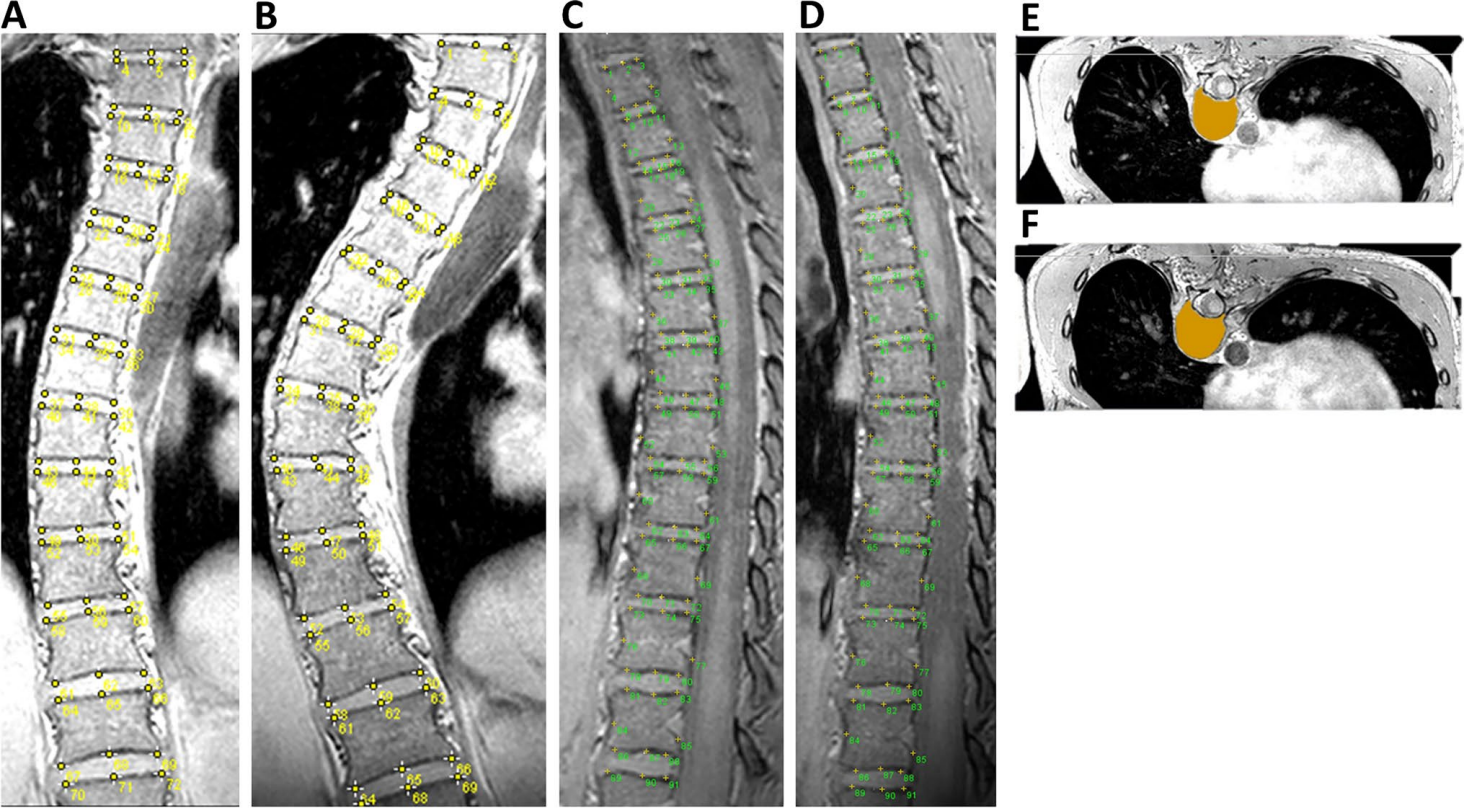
Orthogonal MRI reformatting images from final MRI scans of a non-progressive AIS curve (coronal Cobb angle = 23 deg; major thoracic kyphosis angle = 5 deg; rib hump = 13 deg) (Images A, C and E, respectively), and a progressive AIS curve (coronal Cobb angle = 64 deg; major thoracic kyphosis angle = 13 deg; rib hump = 18 deg) (Images B, D and F, respectively). Images A and B highlight coronal plane segmental anatomic markers. Images C and D highlight sagittal plane segmental anatomic markers. Images E and F highlight axial plane cuts at the curves’ apices, with an overlying annotation of the endplate surface area (blue)
